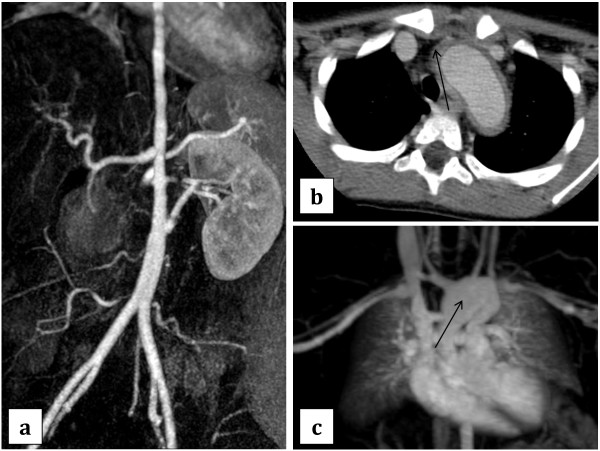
Radiologic abnormalities in children with Takayasu Arteritis. a. Patient 4 per Table 4 with MRA showing significantly decreased caliber and irregularity of the descending thoracic and abdominal aorta, with minimally increased signal characteristics, consistent with inflammatory changes, notably in the proximal descending segment. Patient is status post right sided nephrtectomy with single left renal artery, which appears occluded in its mid segment with patent bypass graft arising from the mid infra-renal abdominal aorta to the left kidney. b. Patient 7 per Table 4 with CTA showing inflammation around the aorta. c. Patient 7 per Table 4 with MRA findings of a mildly prominent proximal ascending aorta, moderately dilated distal ascending aorta, and aneurysmal transverse aortic arch extending into the proximal descending aorta. The largest transverse aortic arch dimension is 30 × 26 mm.
